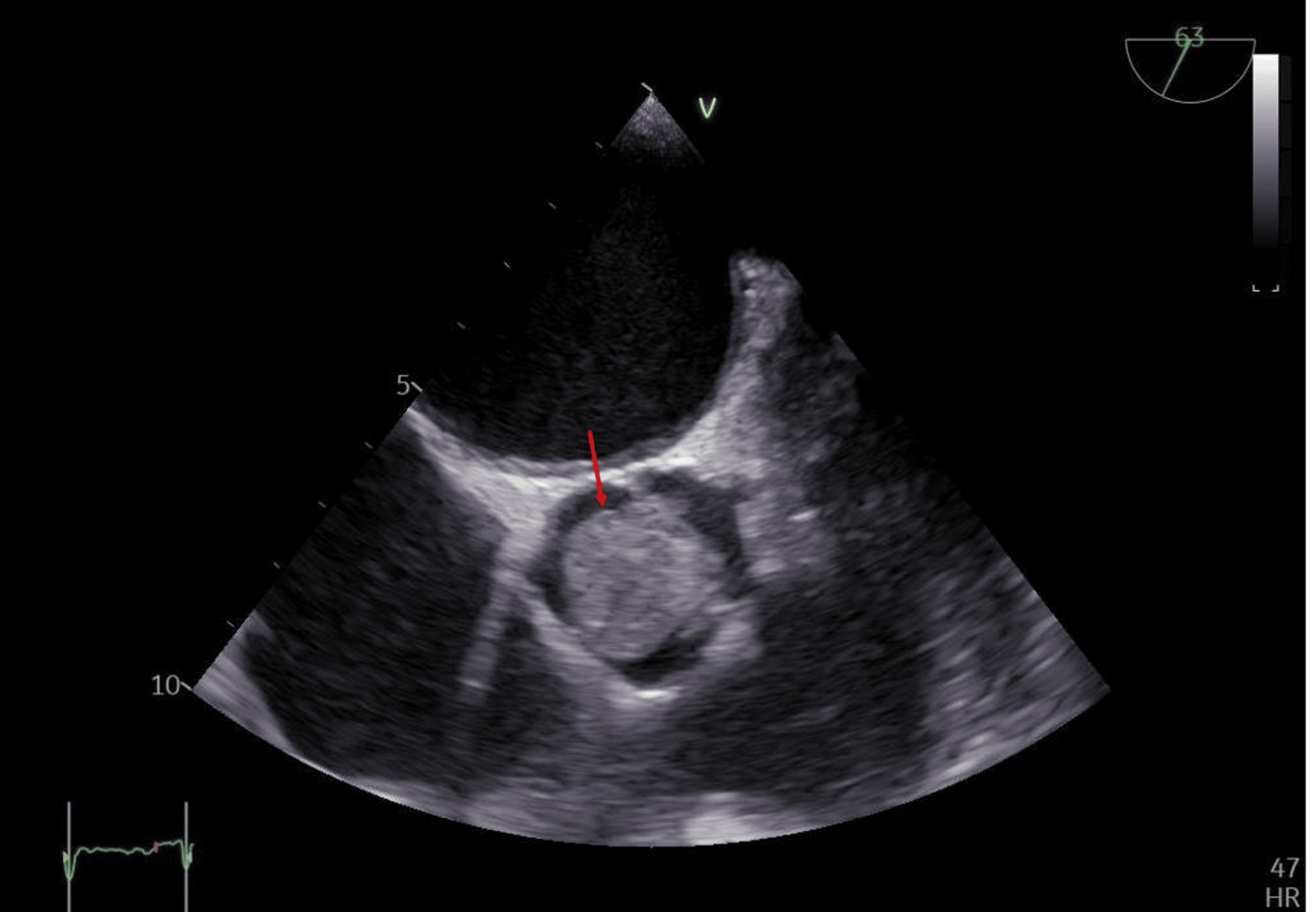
Transesophageal echocardiography (mid-esophageal short axis view) showing the aortic valve mass obstructing the aortic valve orifice, measuring 3.0 x 2.1 cm (red arrow).
